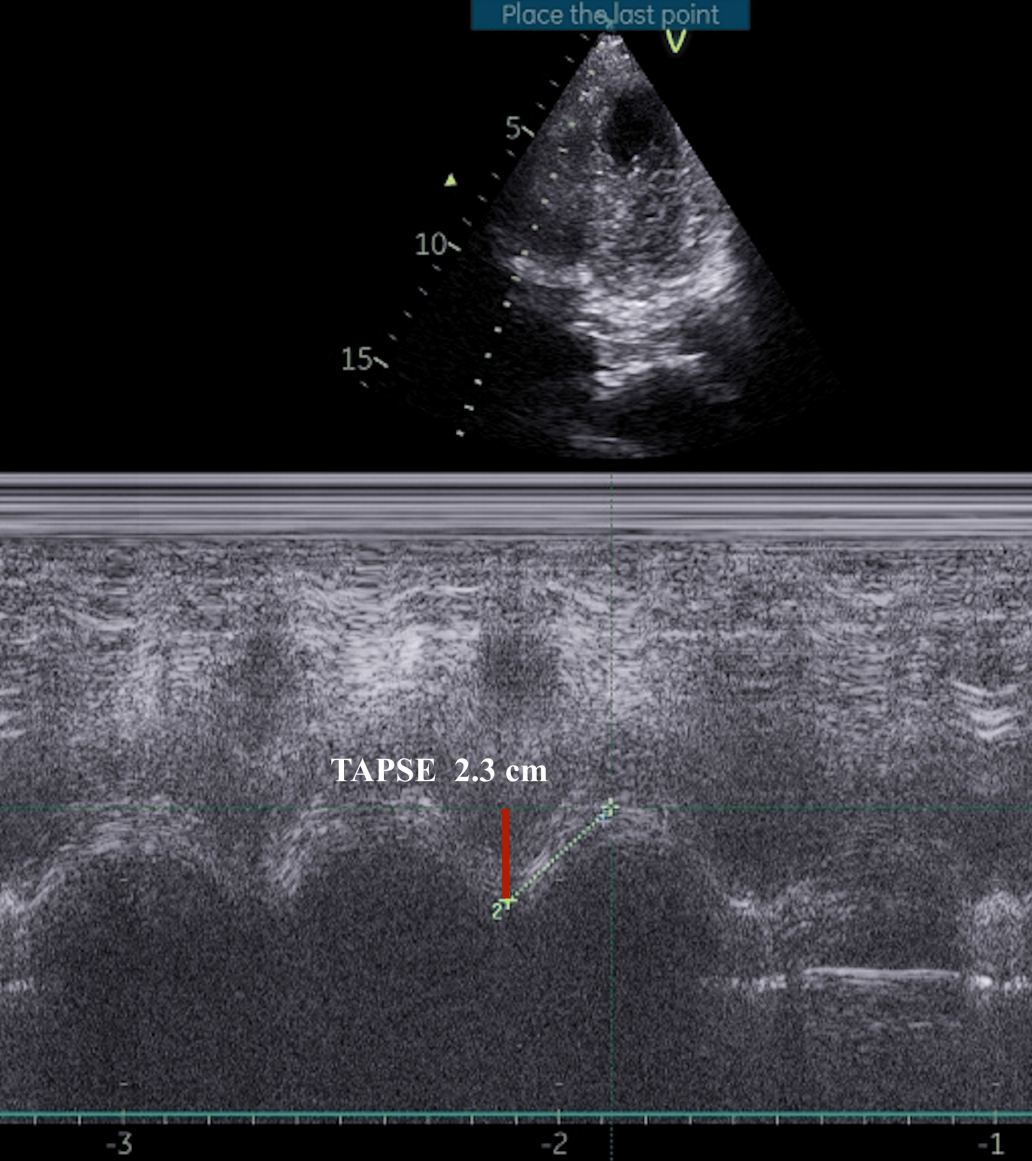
Point-of-care ultrasound derived M-mode echocardiography showing preserved right ventricular systolic function with TAPSE (red line) measurement of 2.3 cm. TAPSE: Tricuspid annular plane systolic excursion.
